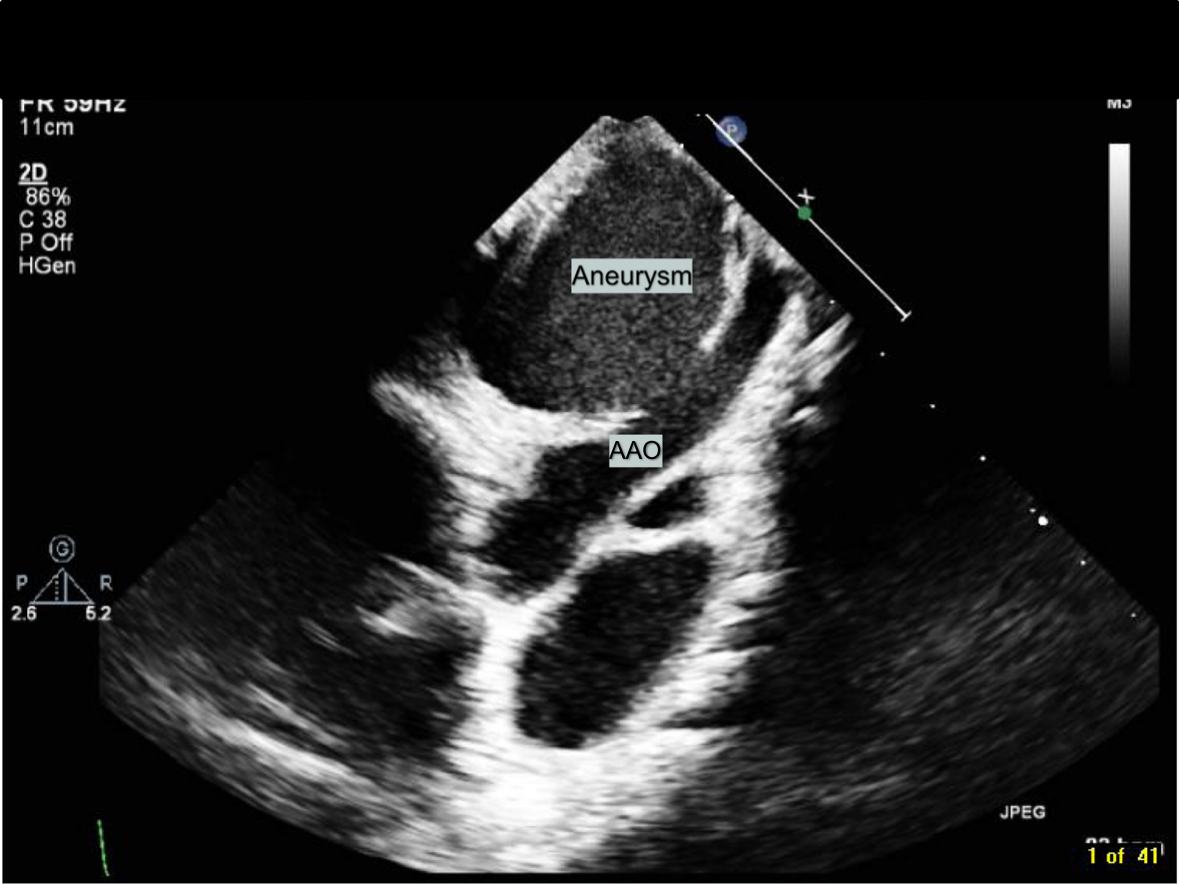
Transthoracic echocardiography, suprasternal view, revealing a huge pseudoaneurysm arising from the anterior wall of the ascending aorta. *AAO* ascending aorta
